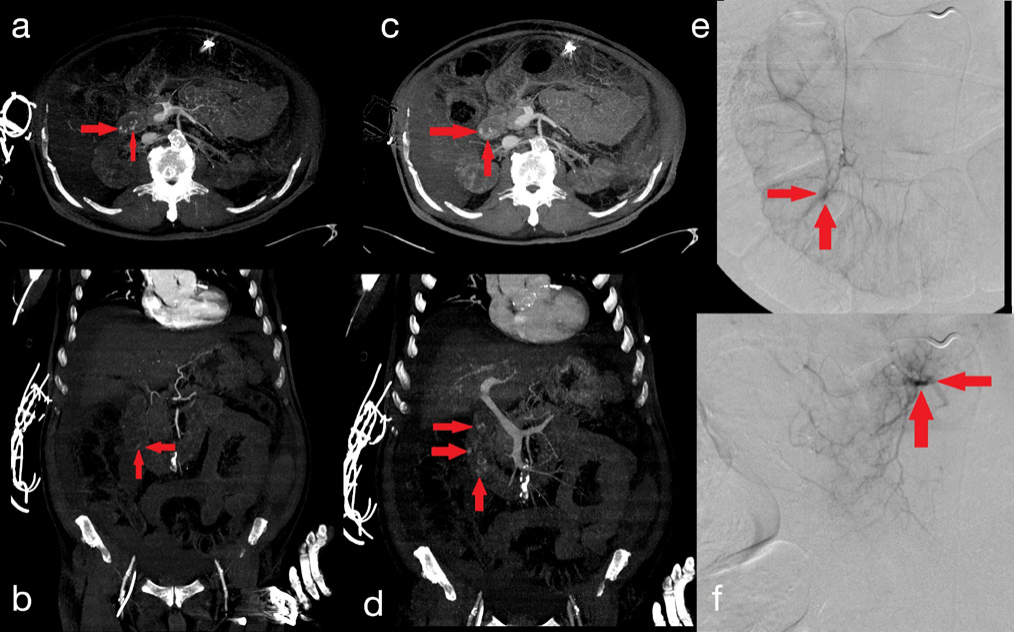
a 79 years old male presenting with recurrent melena, abdominal pain and anaemia. Contrast-enhanced CT scans of the abdomen show an active extravasation of contrast medium within duodenal lumen, incrementing from the arterial (A-B) to the venous phase of the study (C-D; red circles). DSA is thus performed: the presence of an active bleeding from a branch of the gastro-duodenal artery is confirmed (E; red circle) and successful TAE with 2 ml Glubran 60% is performed (F).
